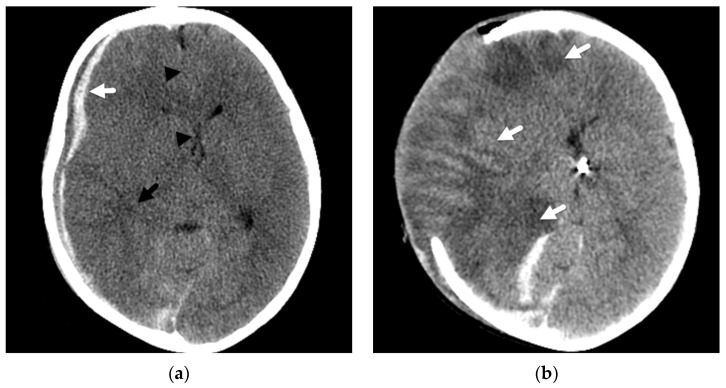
Mixed attenuation right subdural hemorrhage in a 3-year-old female fatality presenting with abrupt mental status change and multifocal bruises after a reported fall. (a) Initial axial noncontrast head CT demonstrates a mixed attenuation subdural hemorrhage (white arrow) with mass effect, midline shift (black arrowhead), and effaced right temporal horn (black arrow); (b) next-day CT image shows changes of a decompressive hemicraniectomy with diffuse, abnormal attenuation of the right cerebral hemisphere (white arrow), which herniates through the cranial defect.
